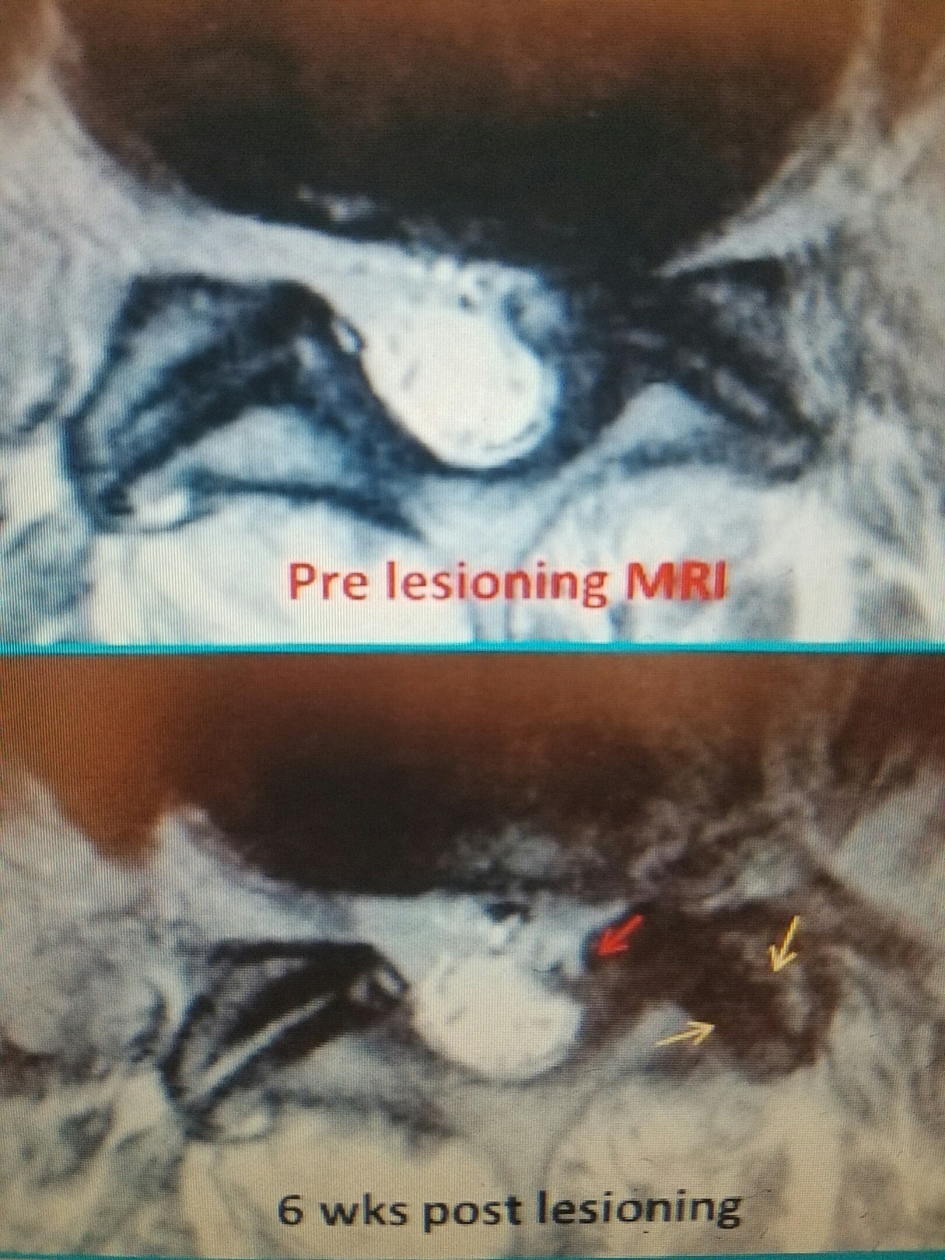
Pre- and postprocedure MRI of L5-S1 facet cyst 81-year-old with radicular pain from L5-S1 facet cyst. Pre-lesioning MRI shows left facet cyst with marked medial foraminal stenosis. Six-week postprocedure MRI shows soft part of cyst signifcantly reduced (red arrow). A small residual 'hook' spur off the medial edge of the inferior facet and less fluid in the facet joint (yellow arrows). MRI: Magnetic resonance imaging.
